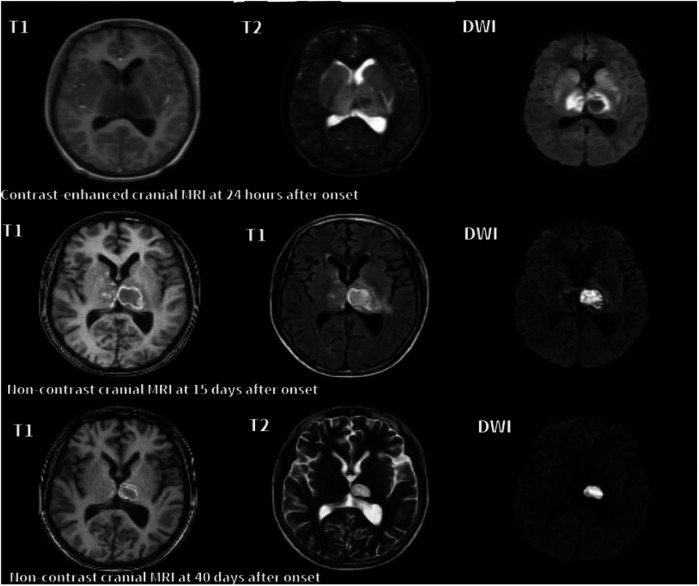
Serial axial MRI images of case 6 with ANE. 24 h after onset: T1 shows mild swelling of the bilateral thalami, while T2 and DWI reveal symmetrical hyperintensities in the thalami, lentiform nuclei, and caudate nuclei, consistent with cytotoxic edema. Marked restricted diffusion is also observed in the thalami. 15 days after onset: T1, T2, and DWI show reduced swelling with heterogeneous signal changes and evolving hemorrhagic transformation in the bilateral thalami and basal ganglia. Persistent restricted diffusion is noted in the right thalamus. 40 days after onset: T1, T2, and DWI show partial resolution of lesions and volume loss in the thalami, indicative of cystic degeneration and encephalomalacia.
